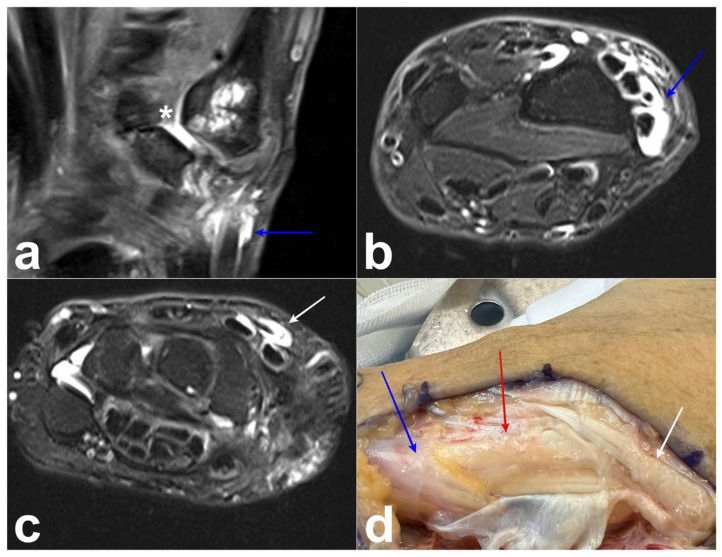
Case 4. (a) Conflict between the first metacarpal and scaphoid (asterisk) and tenosynovitis around the APL tendon (arrow) on MRI (coronal T2-weighted turbo spin-echo with fat saturation and dual-phase acquisition). (b,c) Tenosynovitis of the EPL, ECRB, and ECRL tendons associated with tenosynovitis of the APL and EPB tendons on MRI (axial T2-weighted turbo spin echo with Dixon fat suppression). (d) Preoperative photograph showing tenosynovitis of the EPL (white arrow), ECRB, ECRL (red arrow), and APL (blue arrow).
